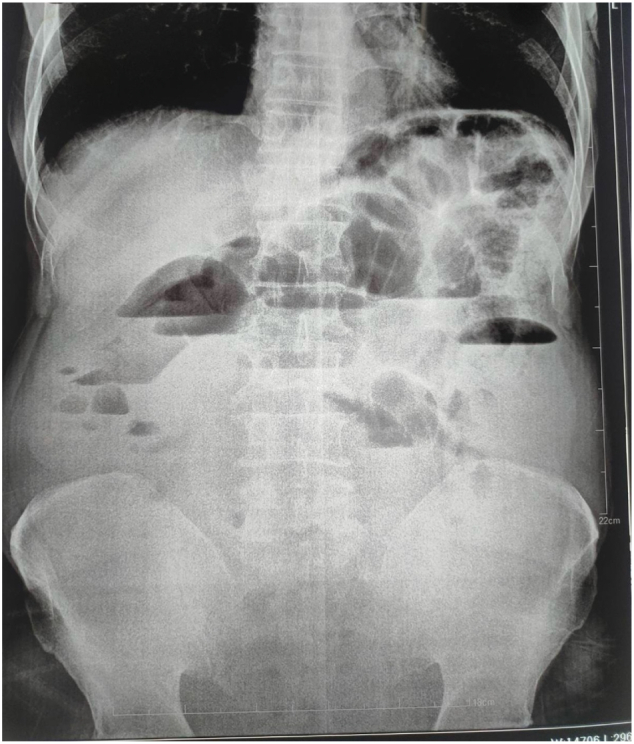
The X-ray abdomen erect view shows multiple air-fluid levels suggesting intestinal obstruction.
